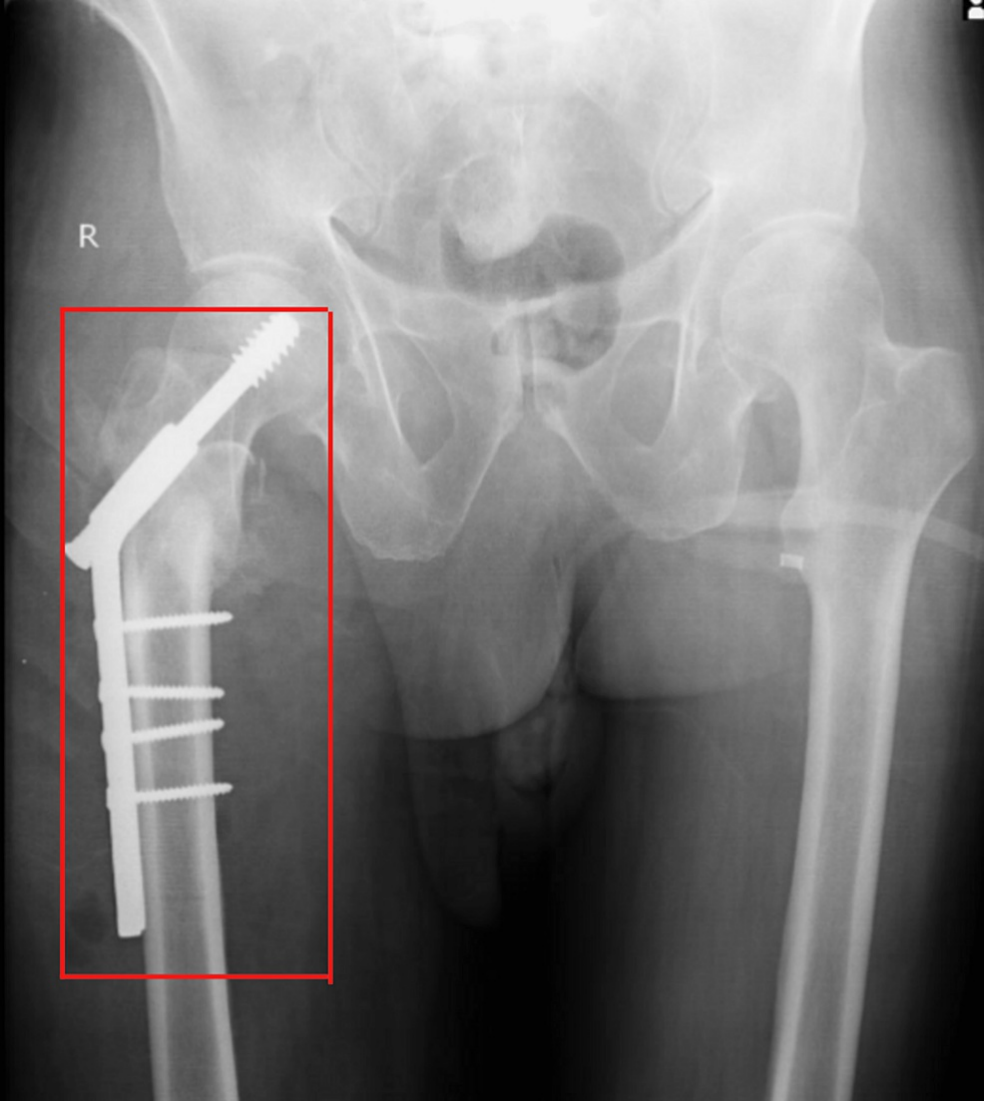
Display an anteroposterior view of an X-ray showing dynamic hip screw fixation of fracture fragments.
